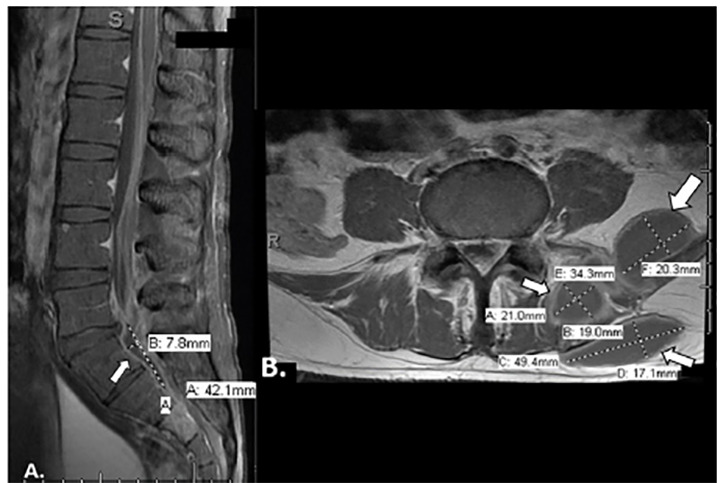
Magnetic resonance imaging of the lumbar spine: A) sagittal view showing an epidural abscess measuring approximately 0.3 × 0.8 × 4.2 centimeters causing severe compression of the thecal sac (white arrows); B) axial view showing a paraspinal abscess at the fourth and fifth lumbar level contiguous with component extending to the subcutaneous soft tissue, and an additional abscess extending inferiorly into the left sacral paraspinal musculature (white arrows).
